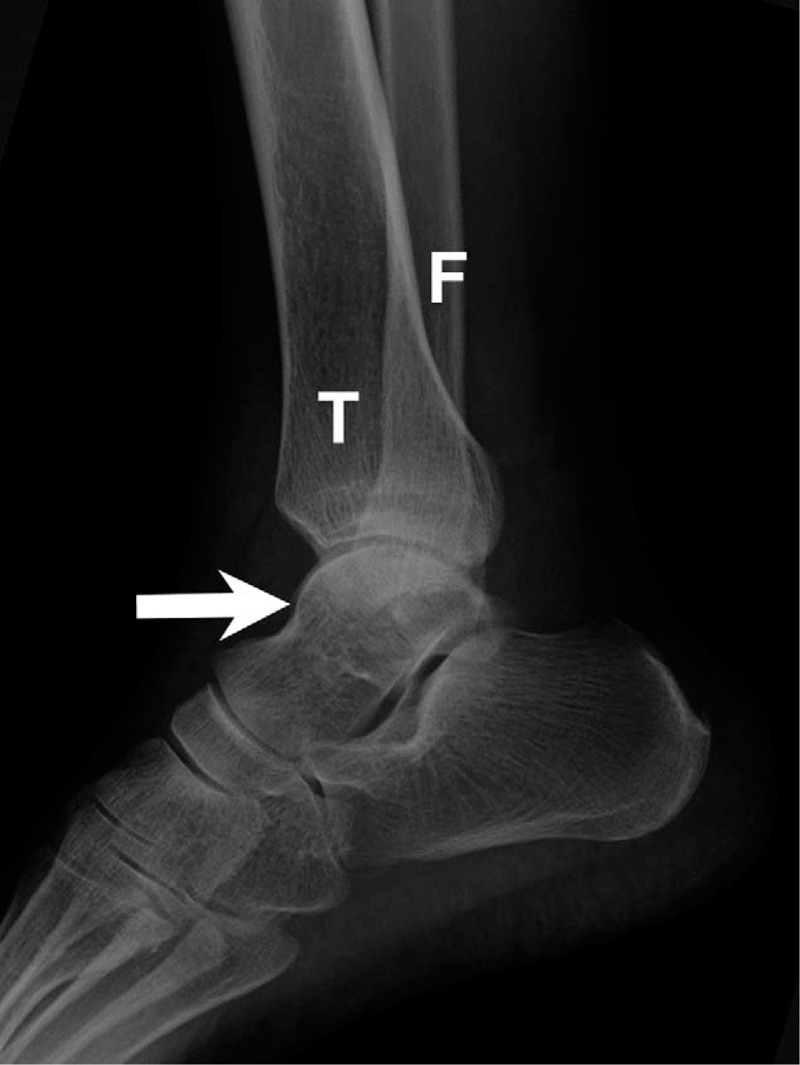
Talar fracture with bimalleolar fracture. Ankle lateral plain film. Arrow = talus, F = fibula, T = tibia.
